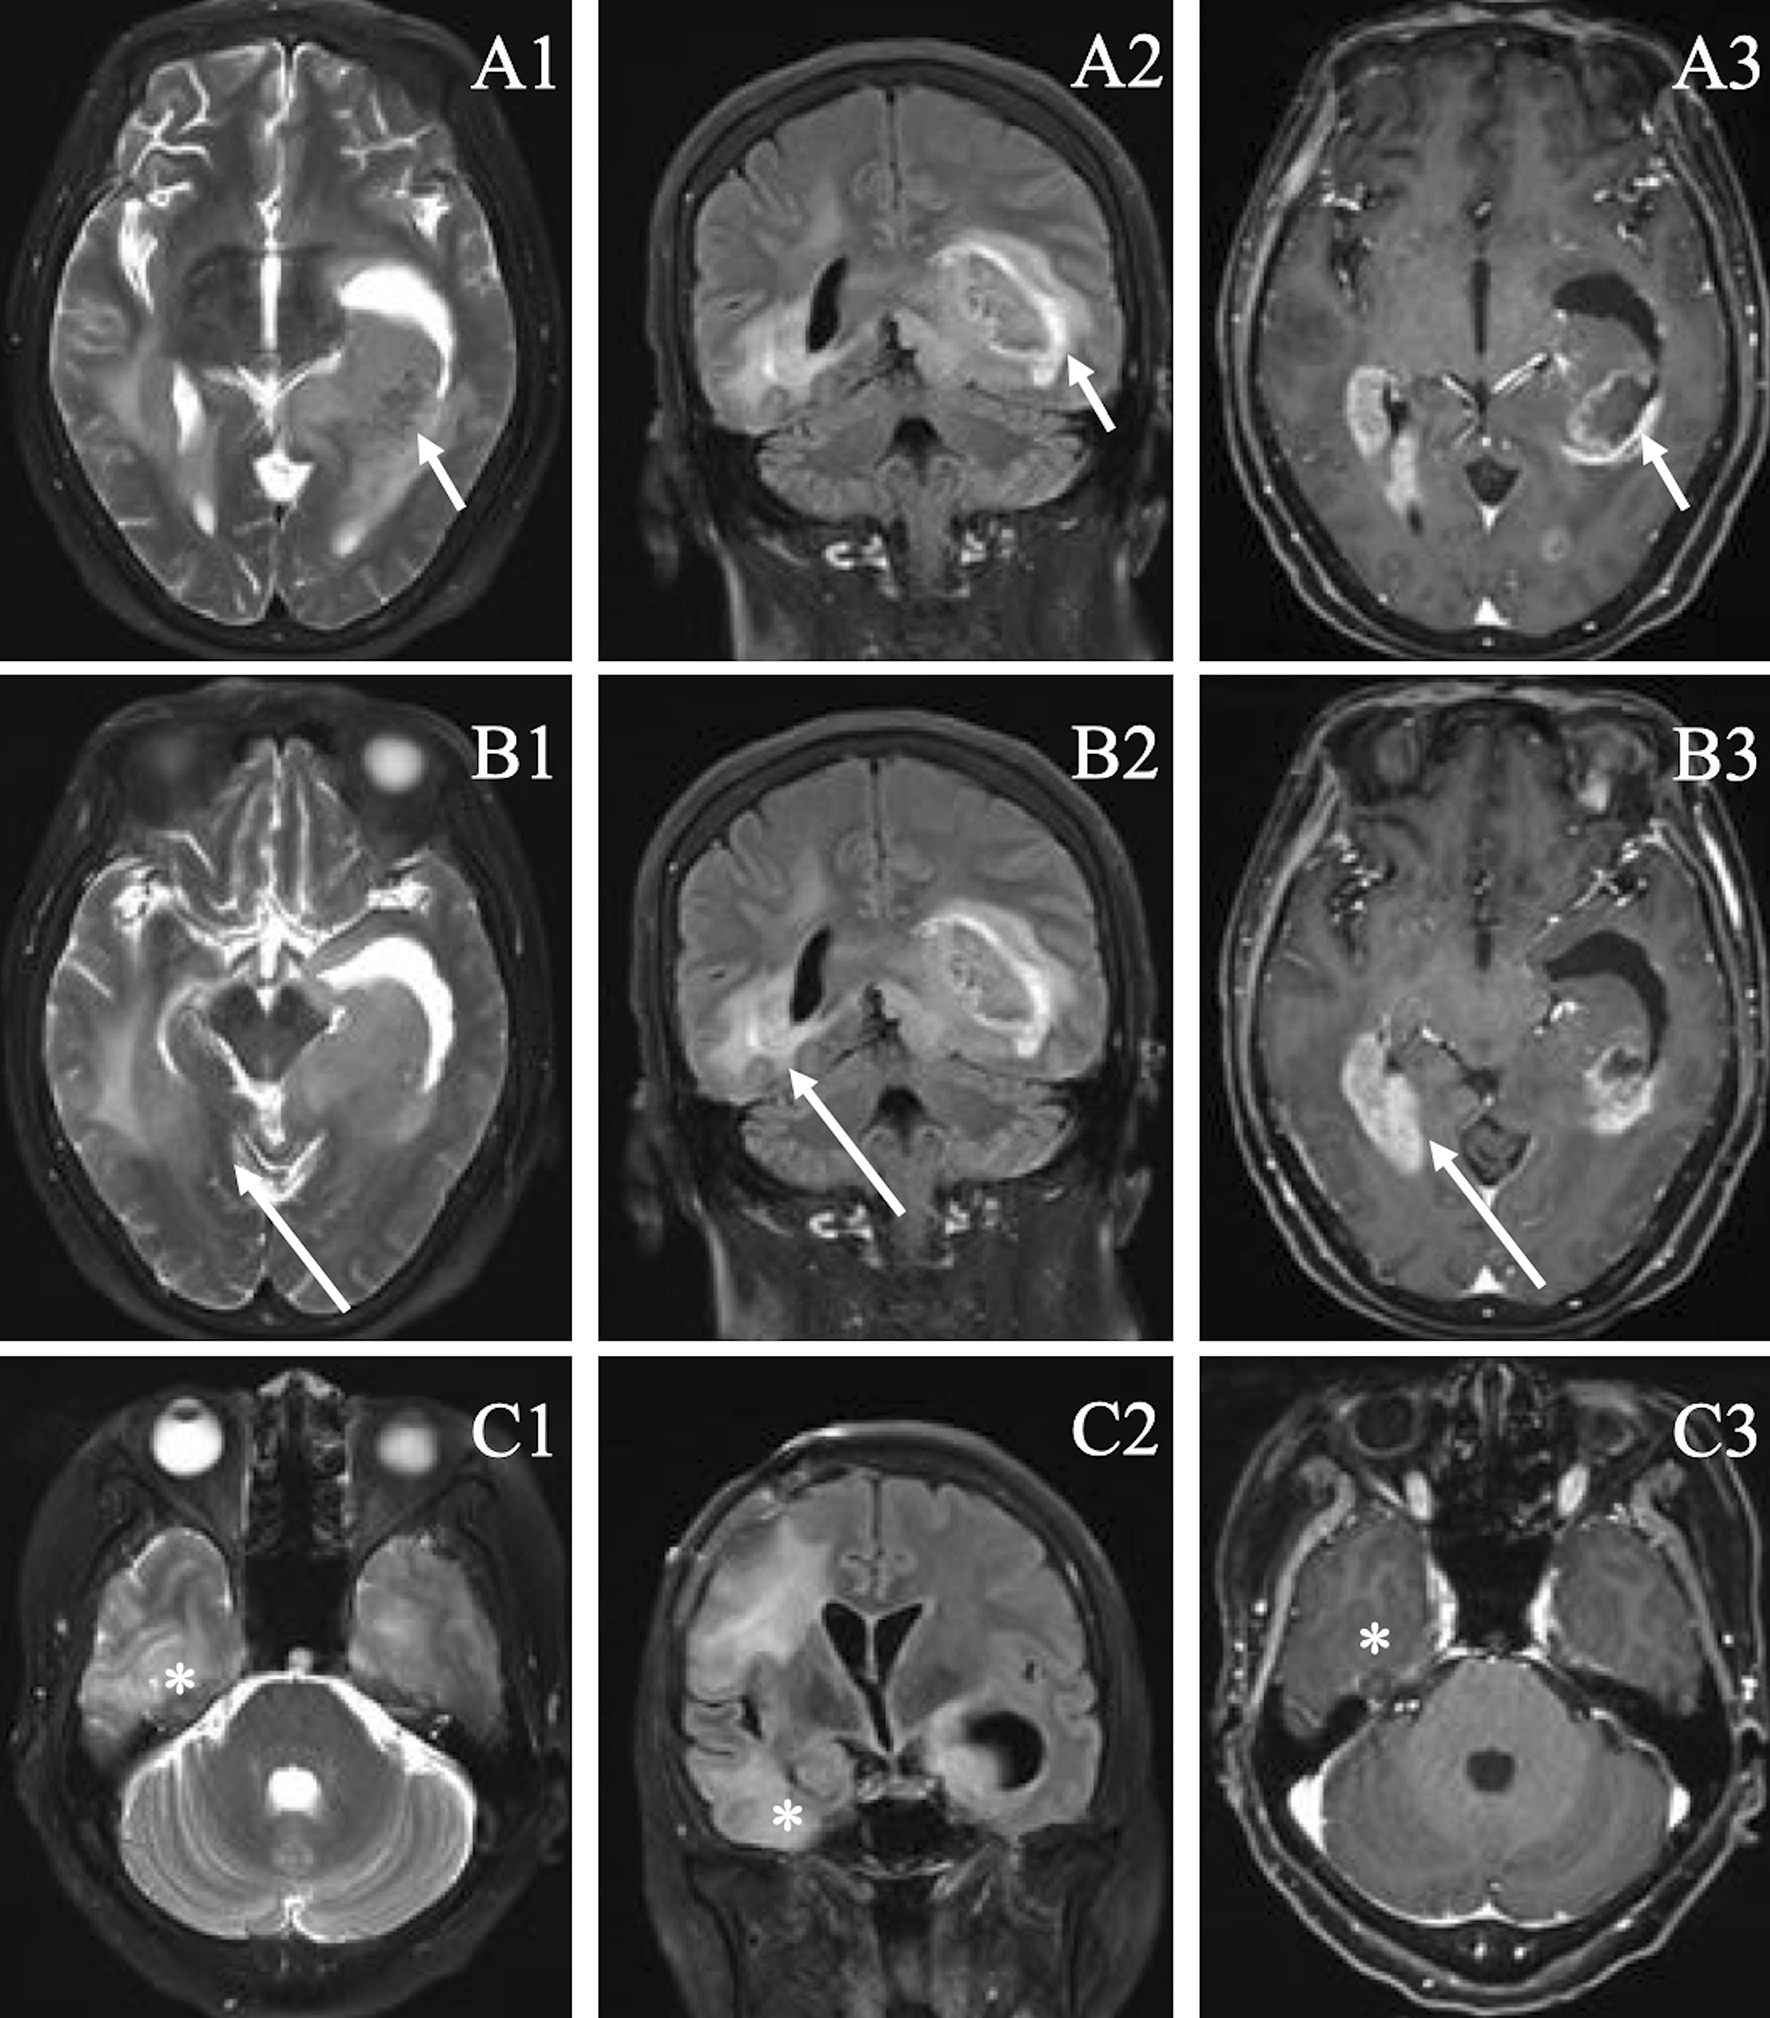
The non-FAPI-avid lesions were confirmed by follow-up MRI (T2, FLAIR, CE-T1) 6 months later. A1–C1 were images of T2 sequence, A2–C2 were images of FLAIR, A3-C3 were images of enhanced T1. All enlarged and partially mixed signals were heterogeneously enhanced (short arrow, long arrow, and asterisk), indicating disease progression
